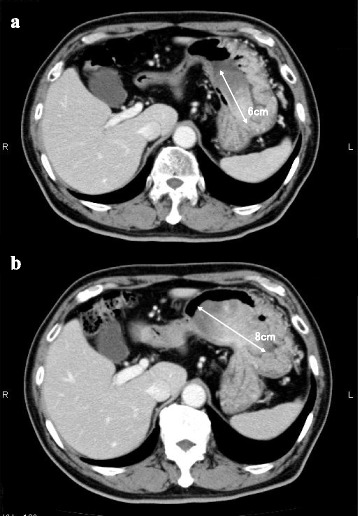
Abdominal computed tomography (CT) showed a 6-cm gastric tumor in early August (a) that had enlarged to 8 cm in diameter by early September (b)
